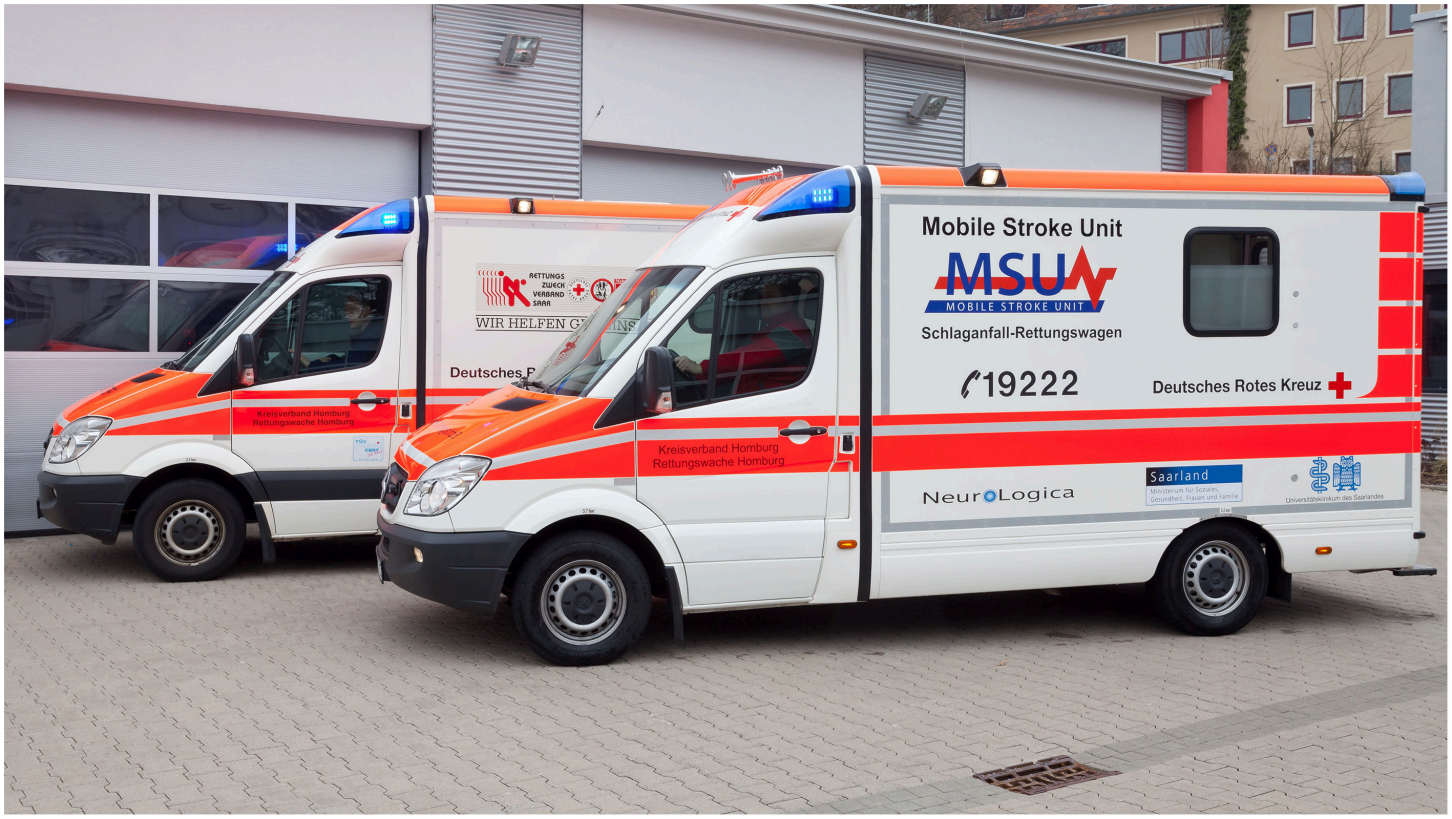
Mobile Stroke Unit (MSU). The Mobile Stroke Unit is an ambulance which contains a multimodal CT scanner, a point-of-care laboratory, as well as a telemedicine system, which allows transfer of CT images and videos of patient examination for input from hospital specialists. Pictured is the MSU in Homburg, Germany.
